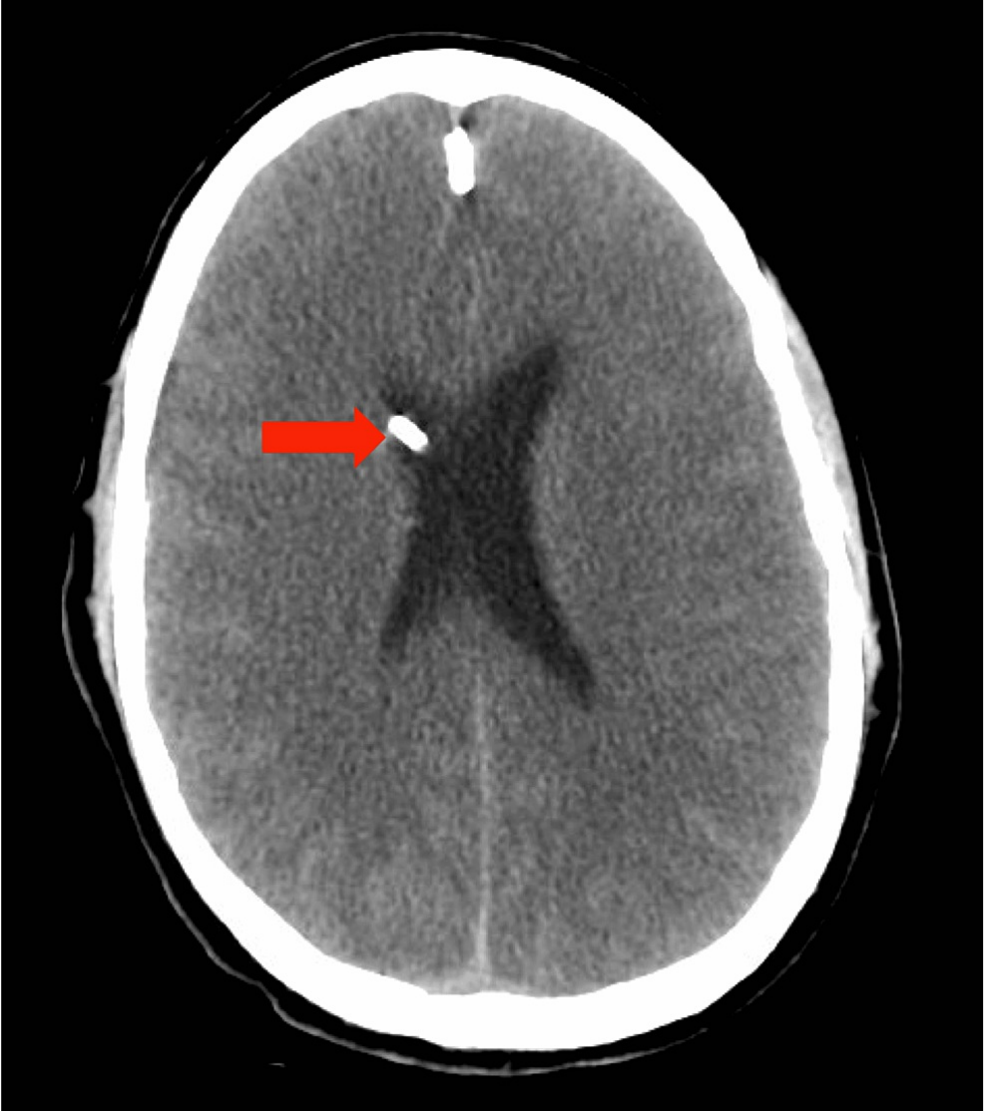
Computed tomography of the head without contrast showing right frontal external vascular drain placement (red arrow). Also evident is diffuse loss of gray/white differentiation.
